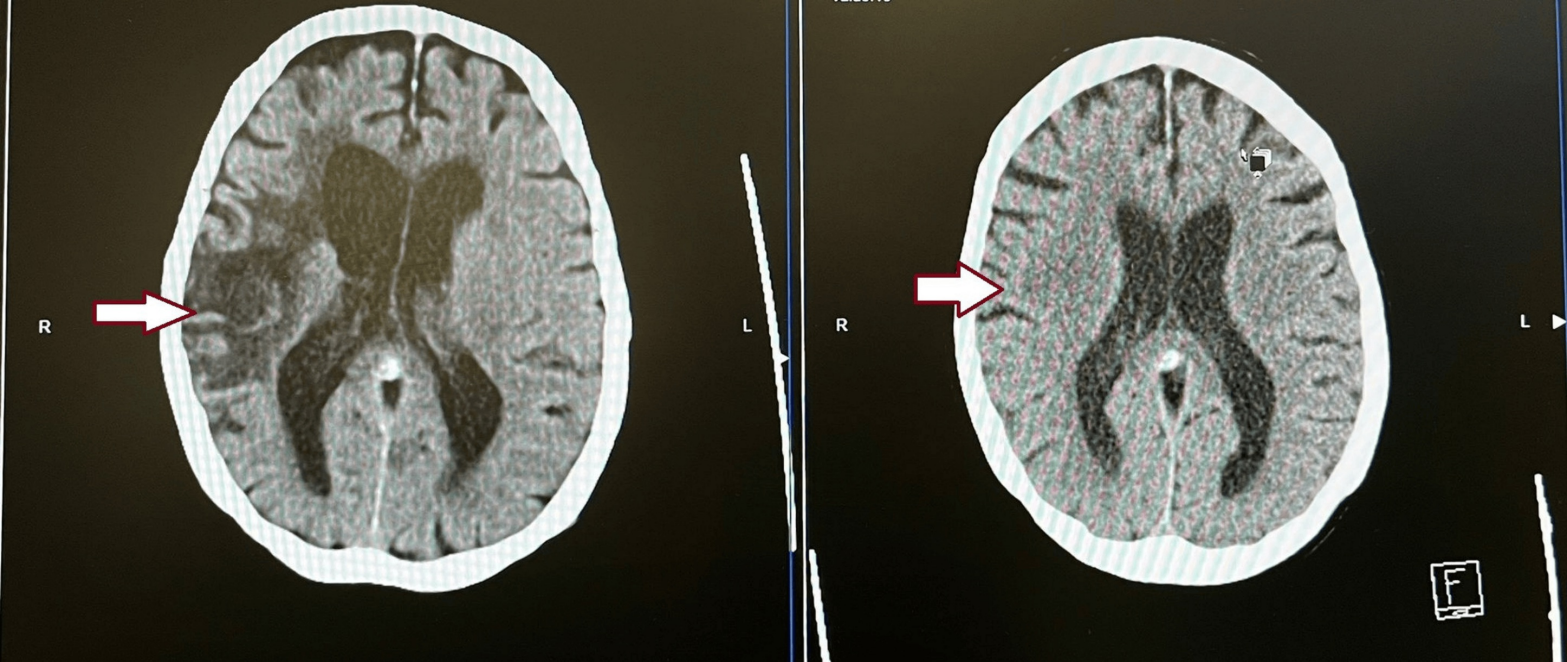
(1a) (2025) well established (chronic) right middle cerebral artery infarction with encephalomalacia which has significantly advanced and new since 2020 CT findings; (1b) (2020) low attenuation affecting grey and white matter in the right frontal lobe in part of the right middle cerebral artery; consistent with acute infarct Arrow points out the extension of infarct from the right frontal region in 2020 to the right temporal, insula and basal nuclei region with brain tissue swelling in 2025.
